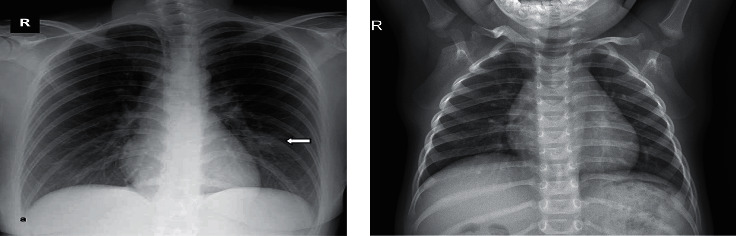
X-ray sample images: (a) COVID-19 X-ray and (b) normal X-ray.
